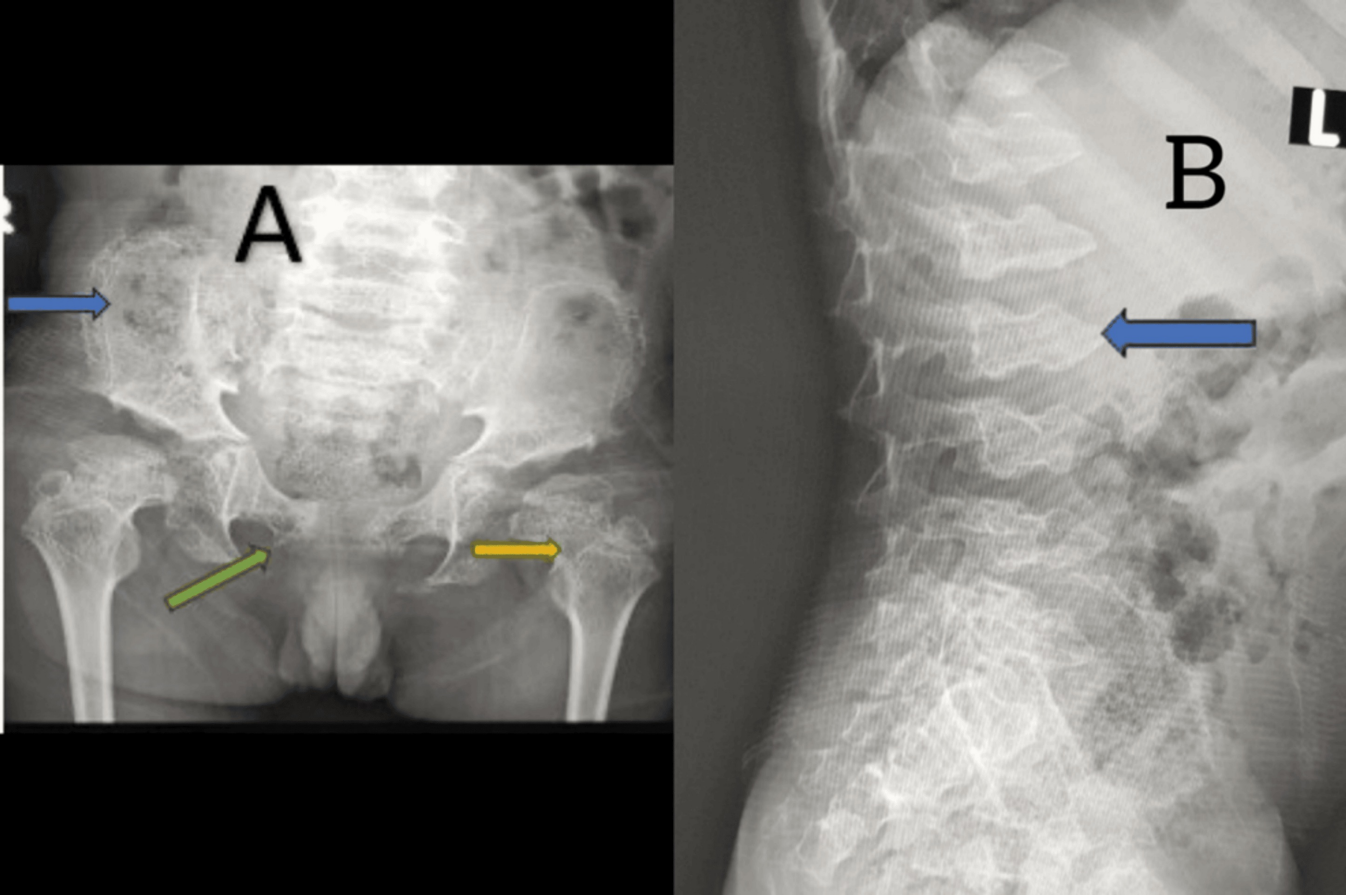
X-ray images A: Anteroposterior X-ray pelvis showed a lacy pattern of the iliac crest (blue arrow), short femoral neck (yellow arrow), and widening of pubic symphysis (green arrow). B: X-ray spine showing anterior peaking and double hump appearance of vertebrae with increased convexity.
